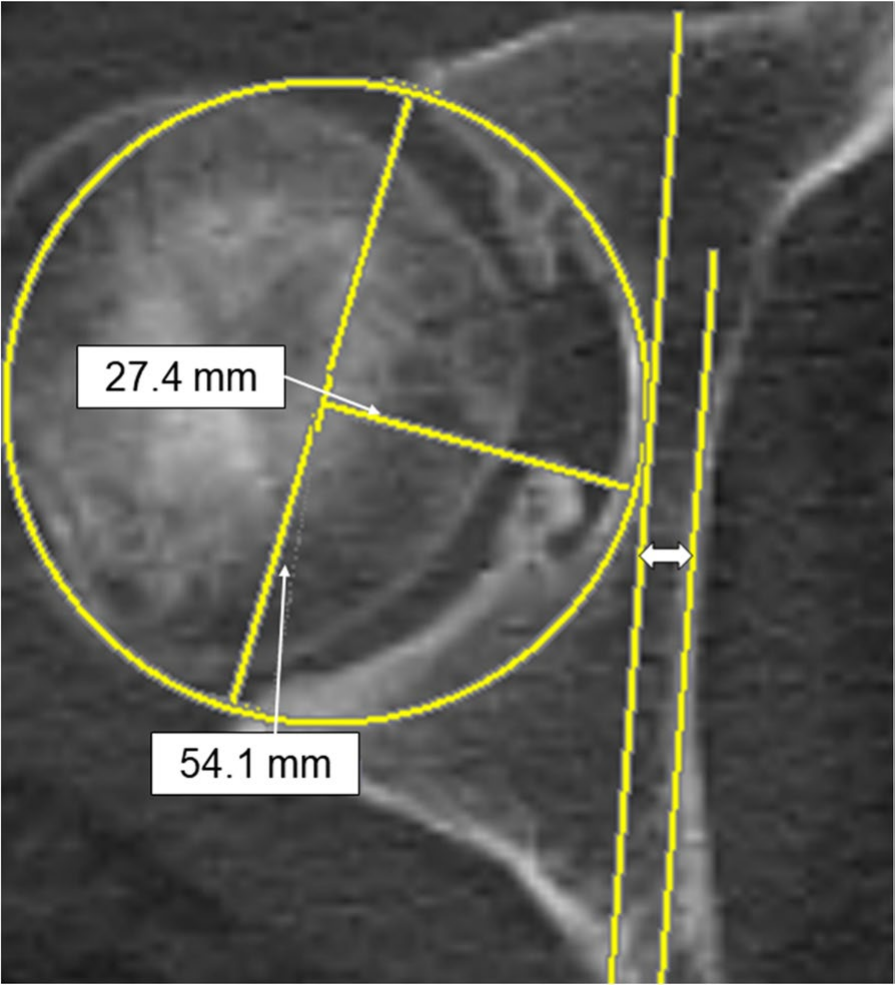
Preoperative planning based on CT images. The slice of the femoral head with the largest AP diameter on CT axial images is selected as the hip center, with the cup diameter estimated by using the distance between the edge of the anterior wall and the edge of the posterior wall of the acetabulum. The depth where the cup is placed is predicted from the radius of the acetabulum. If the position of the ilioischial line on the CT image is known, information concerning the offset of the cup could be obtained. “⇔” indicates the expected distance from the ilioischial line to the cup. CT, computed tomography
